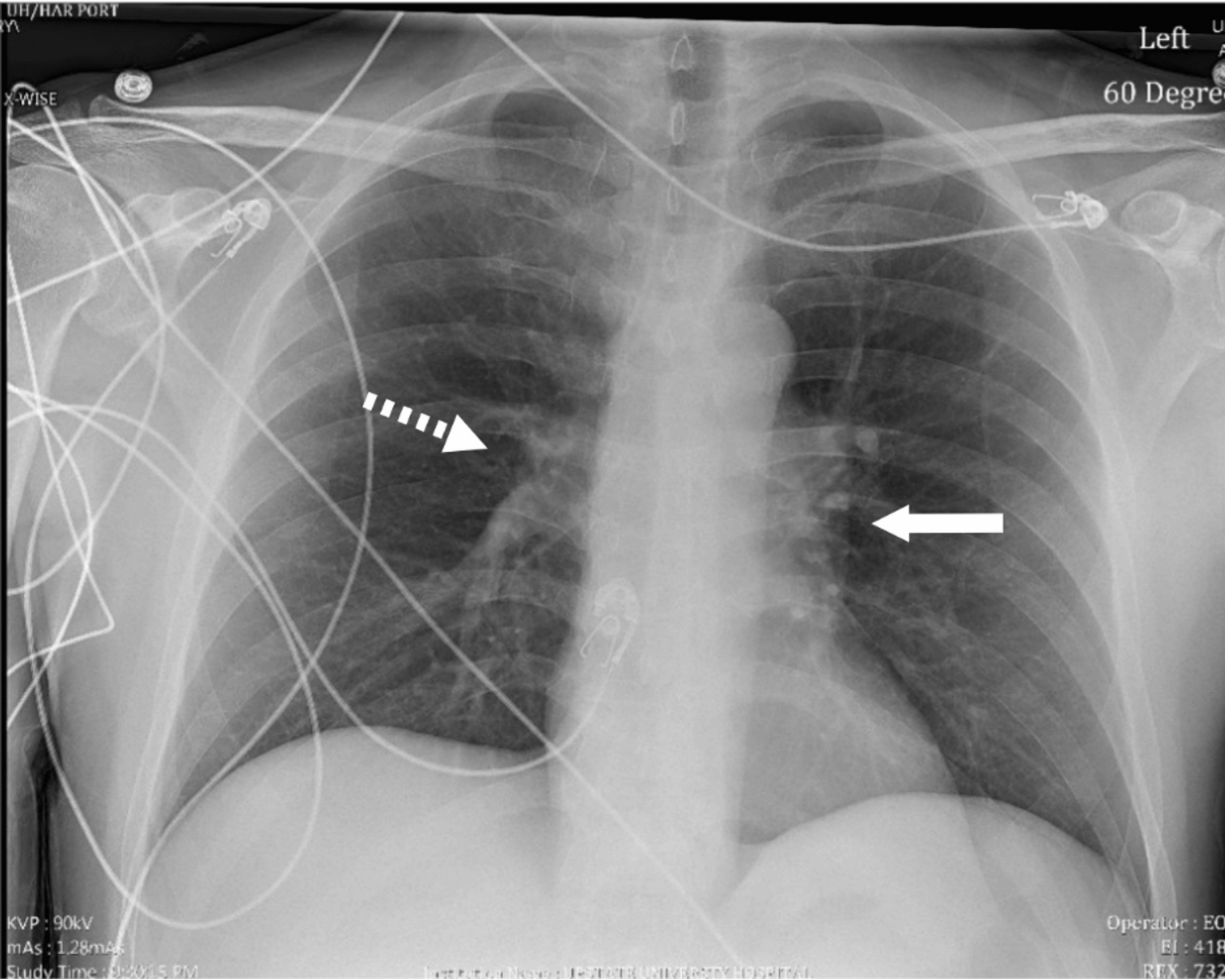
Radiograph of the chest showing left heart border prominence suggestive of enlarged pulmonary trunk (solid arrow) and pulmonary vascular congestion (dashed arrow).
